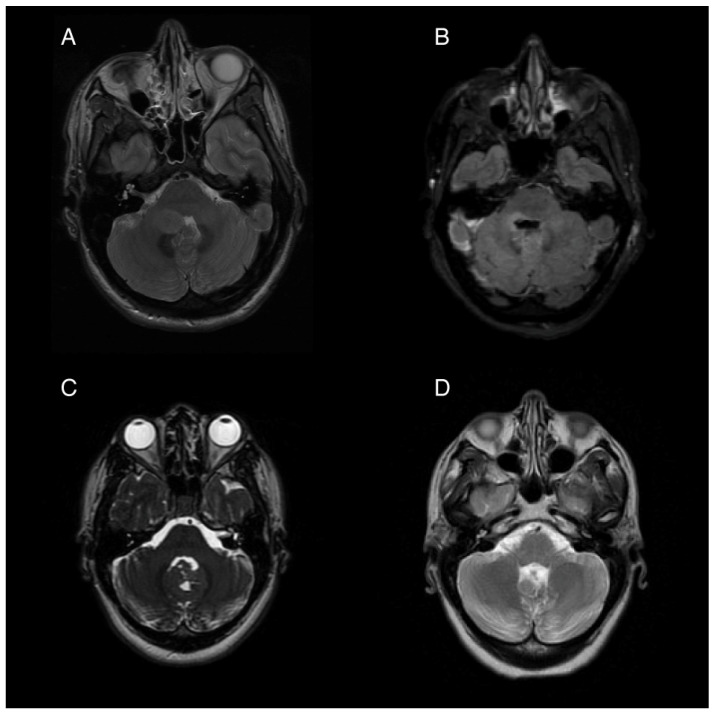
Pre- and post-operative magnetic resonance imaging (MRI) of two patients with posterior fossa neoplasms. (A) Pre-operative T2 sequence of Patient 1, showing a 1.9 × 1.5 cm ovoid mass in the right cerebellar hemisphere extending into the fourth ventricle. (B) Post-operative T2 FLAIR image showing postsurgical changes with pontine and medullary hyperintensity. (C) Pre-operative T2 from Patient 2 showing an iso-intense mass partially obstructing the fourth ventricle and extending from the cerebellar vermis. (D) Post-operative T2 showing postsurgical volume loss of the inferior vermis.
